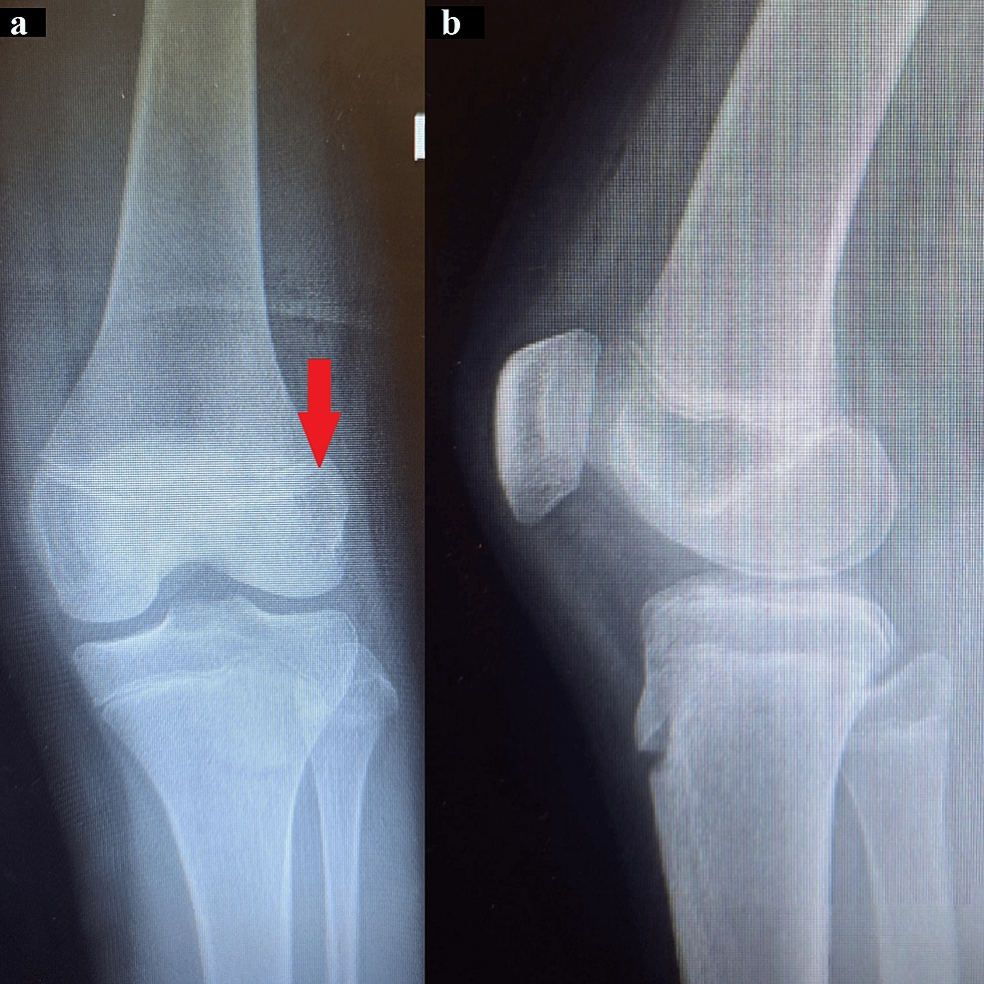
The X-rays were inconclusive, without a clear sign of an intra-articular lesion from the lateral femoral condyle. (a) Anteroposterior X-ray of the patient's affected knee shows the lateral femoral condyle appearing normal. (b) Lateral X-ray of the patient's injured knee reveals no evidence of bone fragments.
